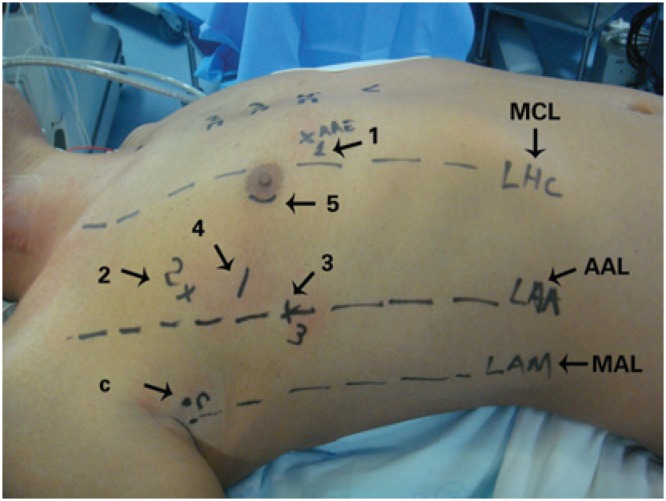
Preoperative markings and sites for the introduction of (1) left atrial retractor; (2) left robotic arm; (3) right robotic arm, (4) working trocar; (5) micro camera. C: transthoracic aortic clamp; MCL: mid-clavicular line; AAL: anterior axillary line; MAL: mid-axillary line
